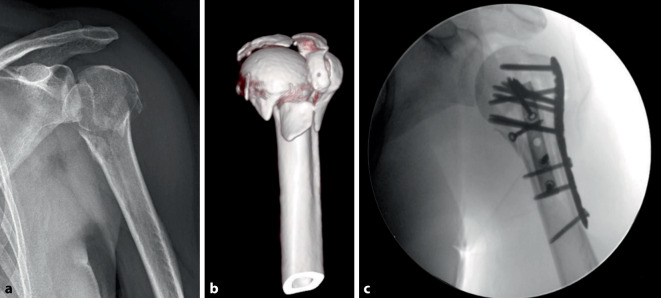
Aufnahmen einer 52-jährigen Patientin mit posteriorer mehrfragmentärer Luxationsfraktur Typ 5 nach Resch et al. [22]. a Präoperatives Röntgenbild, b Volumenrekonstruktion mithilfe der CT-Bildgebung (Ansicht von anterior), c intraoperatives Abschlussbild nach Doppelplattenosteosynthese in Kombination mit autologem Fibula-Graft und freier Schraubenosteosynthese
